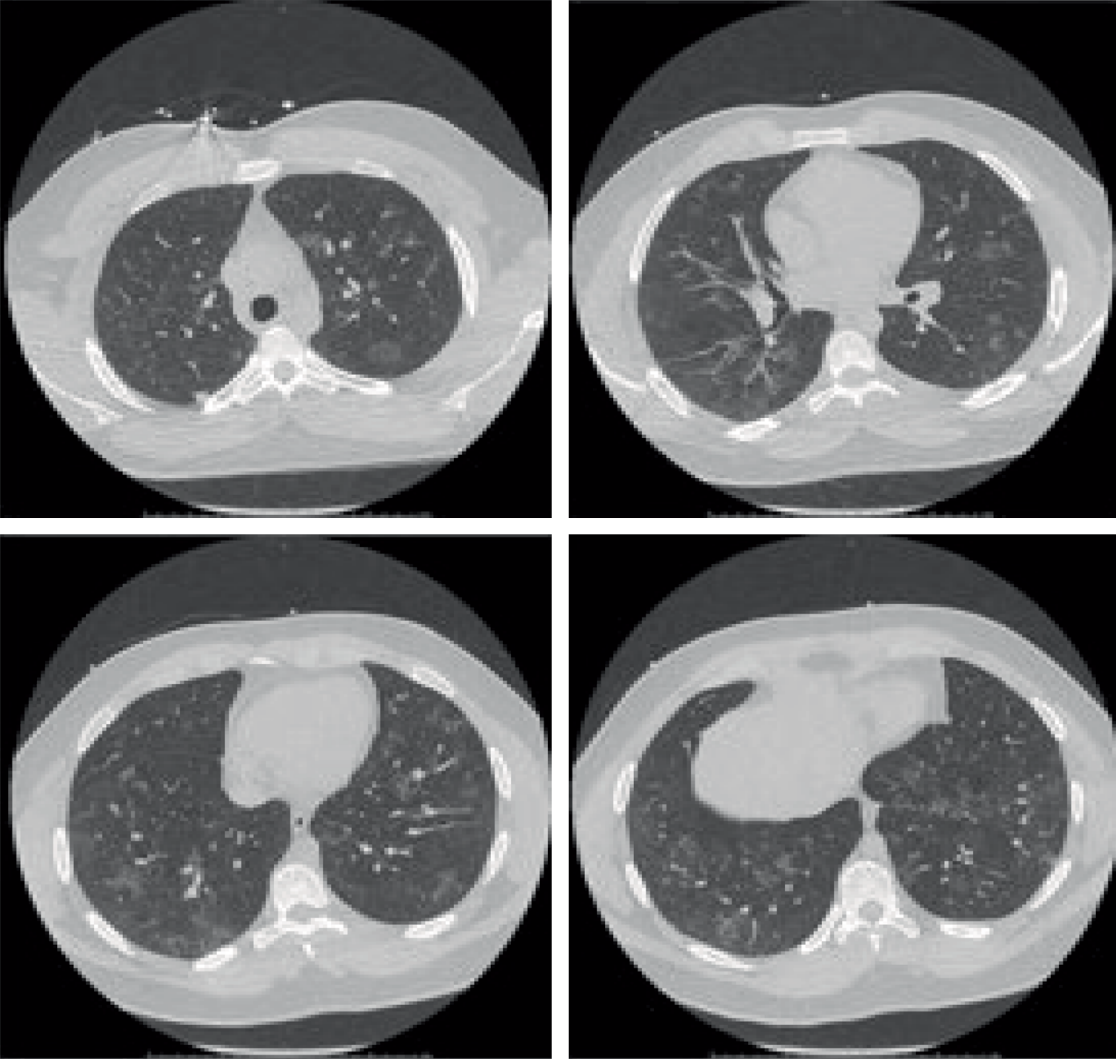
HRCT showing multifocal ill-defined ground glass opacities throughout both lungs.
